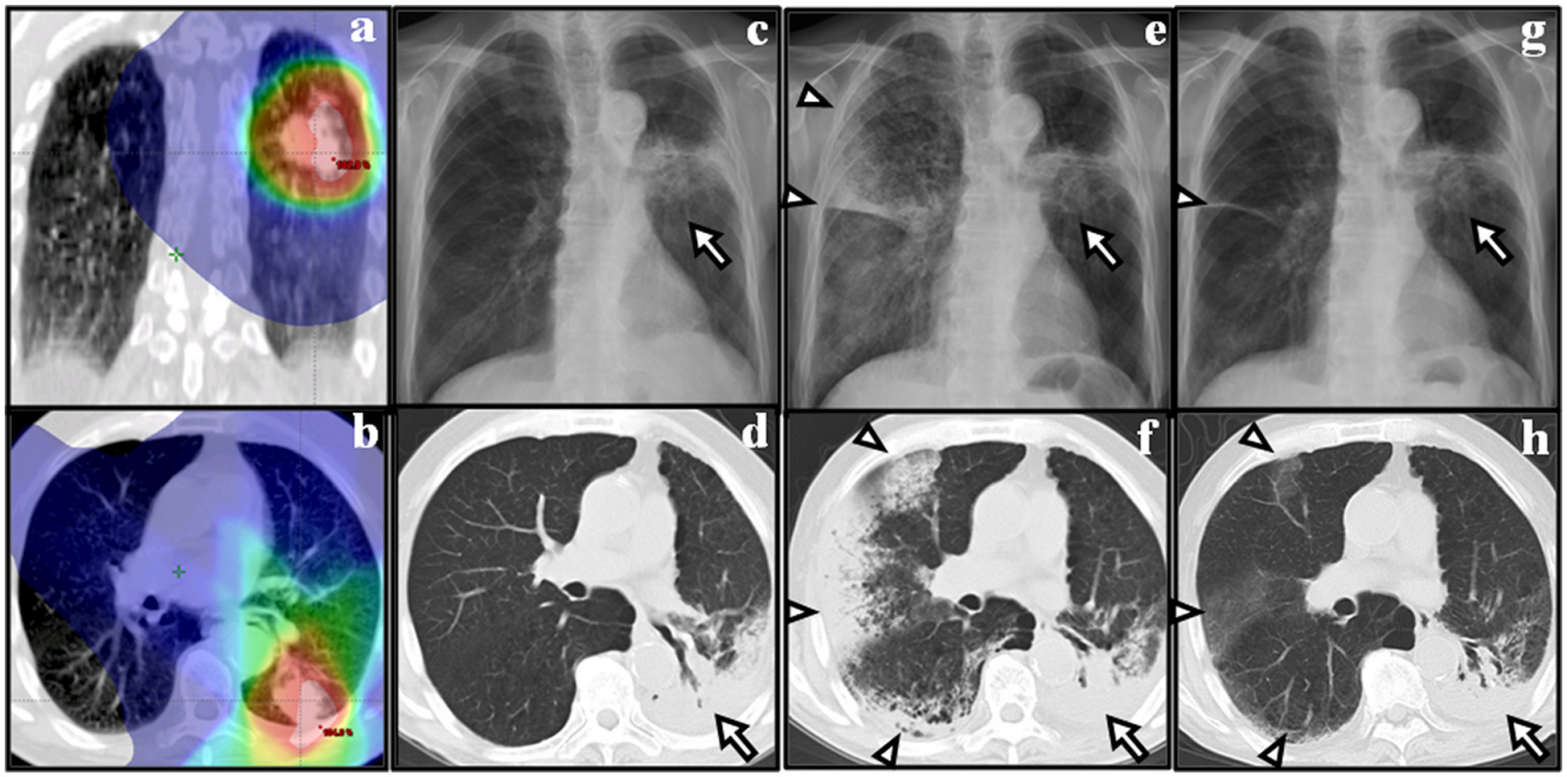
Chest CT, radiograph and dose distribution of SABR of an 82-year-old patient. Blue represents areas receiving more than 0.5 Gy (Figure
